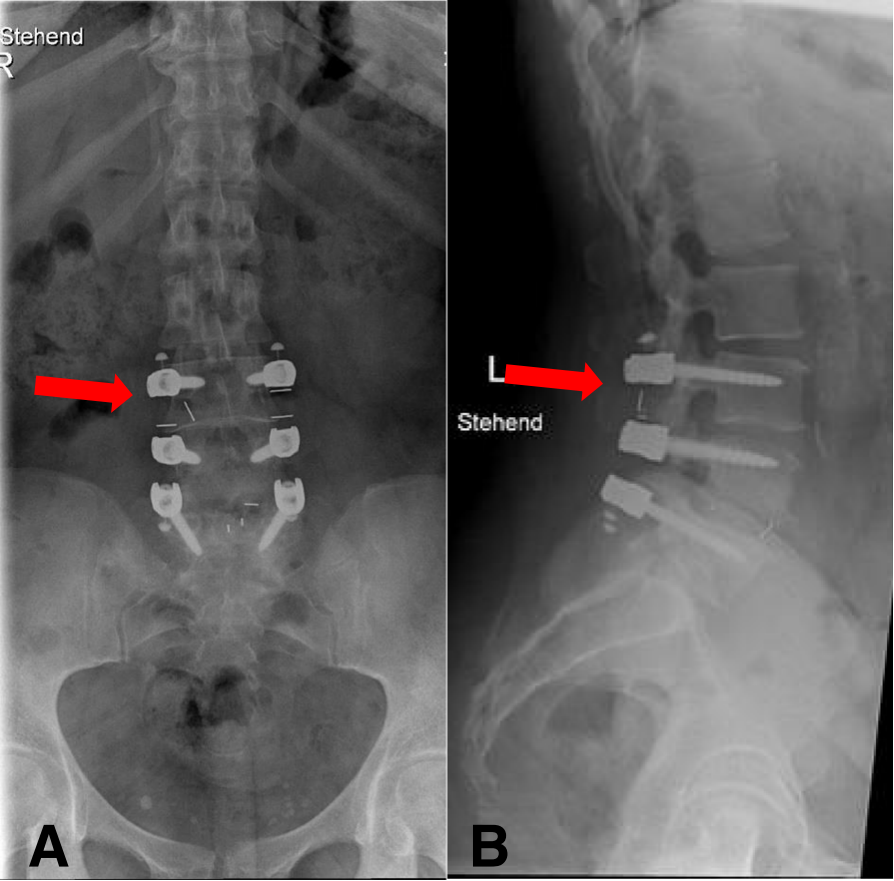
Lumbar spine anterior–posterior (a) and lateral (b) radiographs at the 2-year FU. The red marker shows breakage of the PEEK and silicone C-shaped dynamic part at segment L3–4
